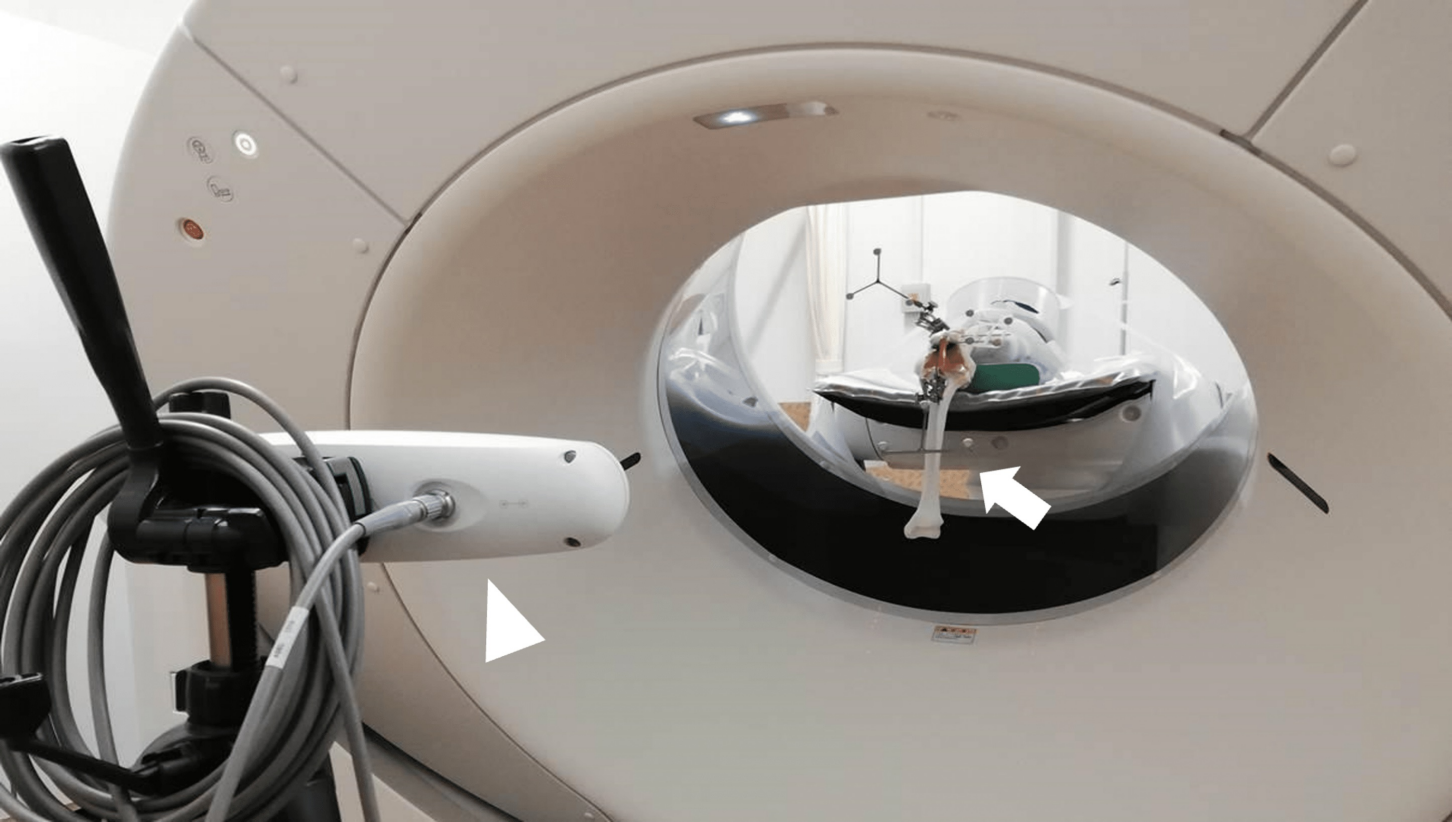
Knee joint model in the CT gantry and the optical-motion capture system The knee joint model was fixed on the CT gantry (arrow), and the optical-motion capture system was placed facing the knee joint model (arrowhead).
